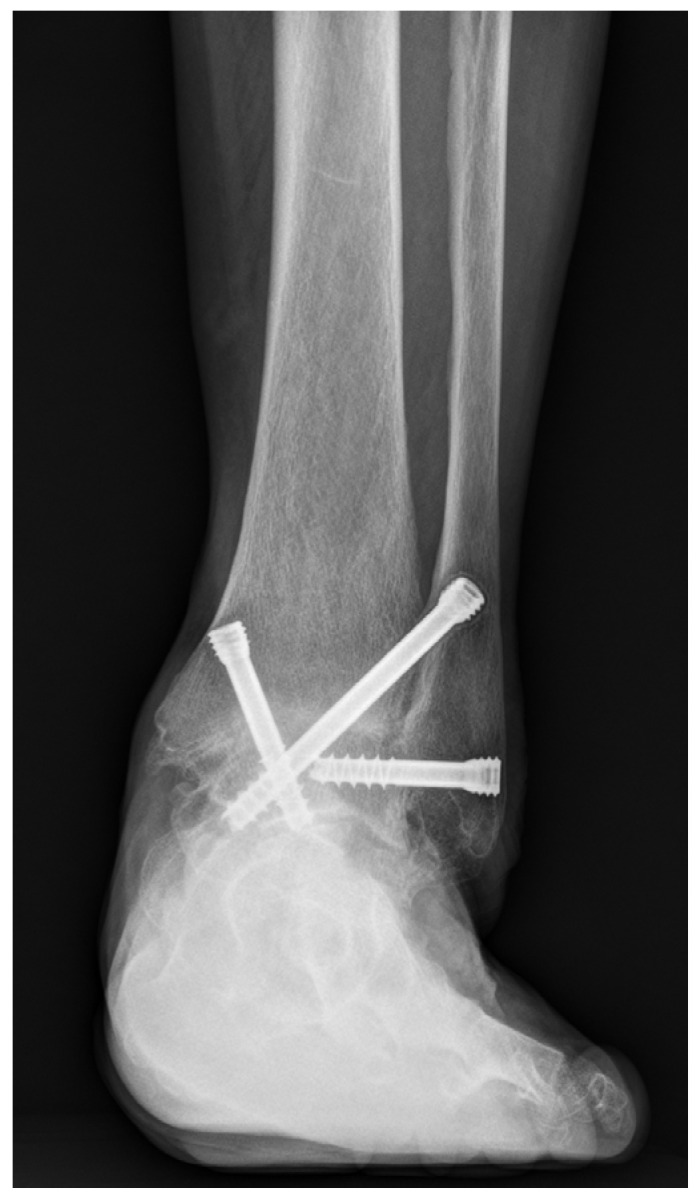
Six-month follow-up plain radiographs demonstrating complete bony union along the arthrodesis site.
